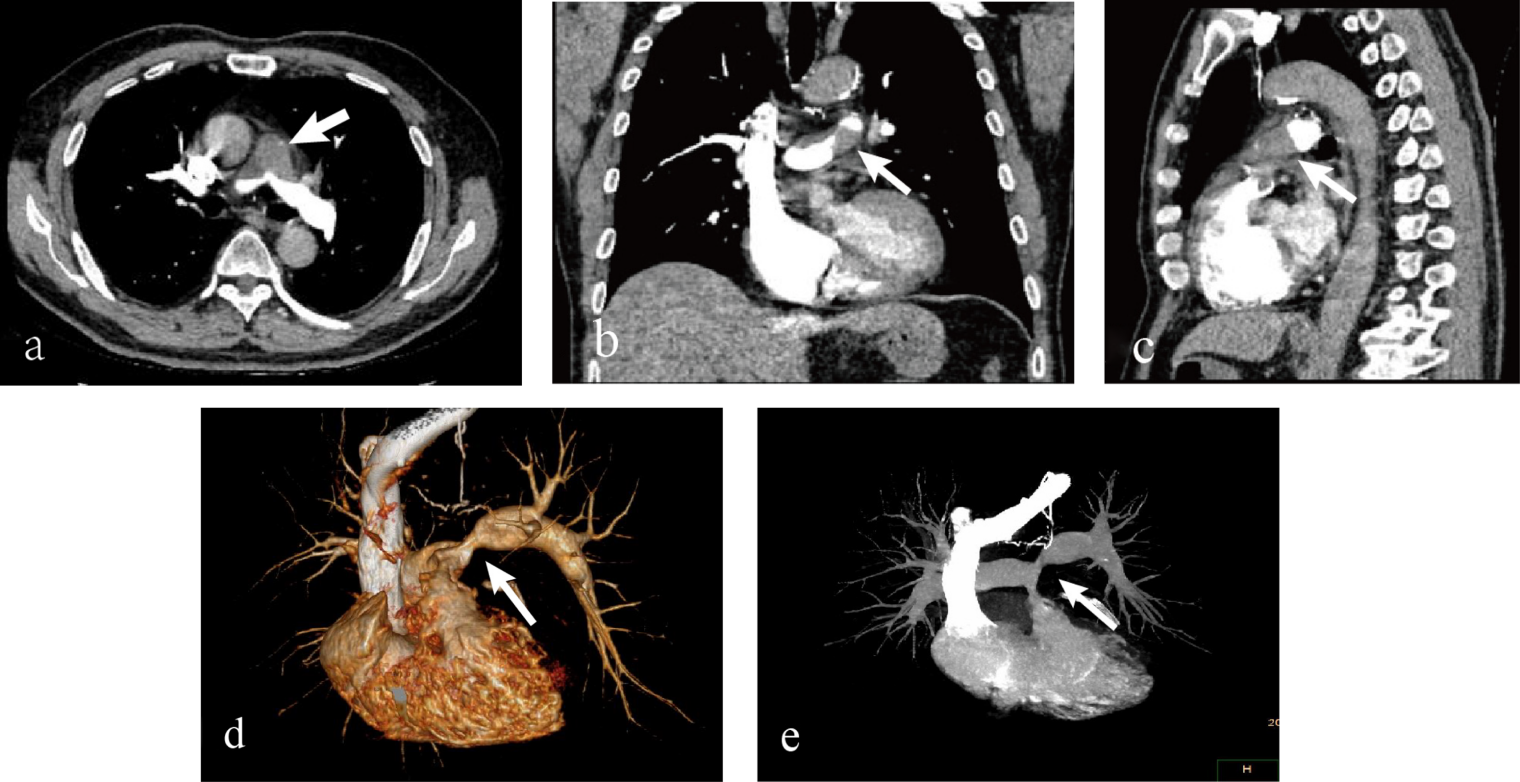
Computed tomography angiography (CTA) findings. (a) Axial contrast-enhanced CT image reveals a well-defined filling defect (arrow) occupying most of the main pulmonary artery lumen. (b) Coronal reformatted image better demonstrates the extent of the mass and its irregular, lobulated border (arrows). (c) Sagittal view further confirms the presence of the filling defect (arrow) within the pulmonary artery. The multi-planar reconstruction comprehensively delineates the lesion. (d) Three-dimensional volume-rendered image of the mass and pulmonary vasculature. (e) Maximum intensity projection (MIP) image highlighting the filling defect within the contrast-opacified pulmonary artery.
